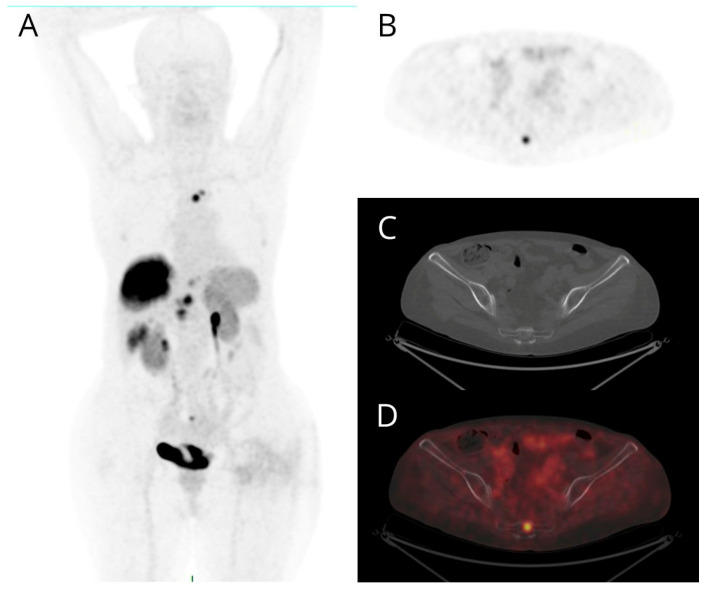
Maximum intensity projection (MIP) (A), PET (B), non-diagnostic CT (C), and fused PET/CT (D) transaxial images of a patient with metastatic G2 pancreatic NET. Bone lesions exhibit intense [68Ga]Ga-DOTANOC uptake (B,D) without morphologically evident corresponding lesion on non-diagnostic CT (C). The other PET-positive findings (A) are two pancreatic nodules, a voluminous hepatic lesion, and further smaller lesions in the VI segment and in the left lobe of the liver, a bone lesion of the sternum and a mediastinal node.
